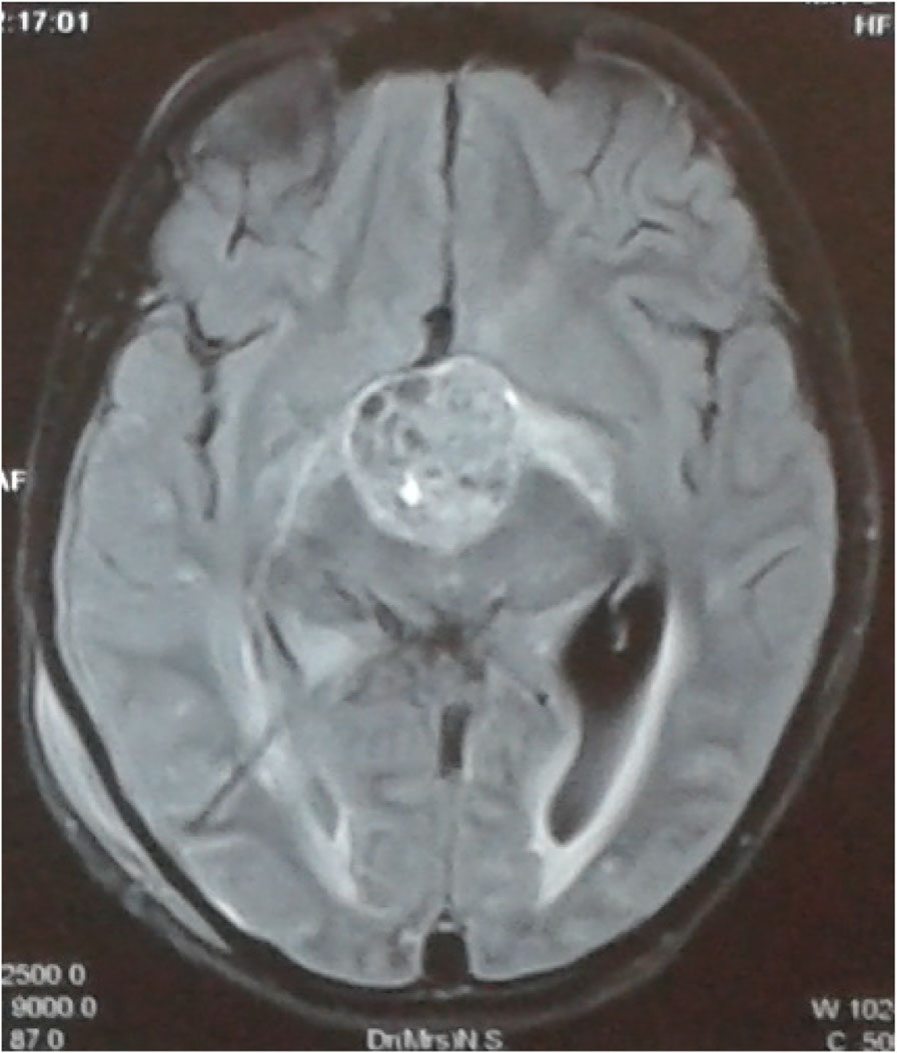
Magnetic resonance imaging T1-weighted pre-contrast axial image showing suprasellar mass suggestive of craniopharyngioma
